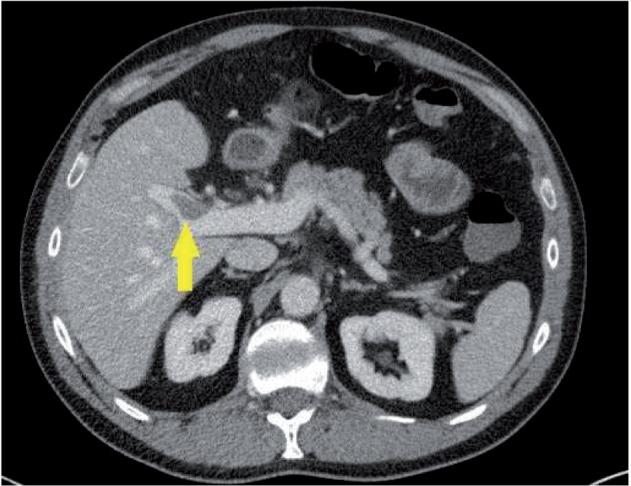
CT of the abdomen, demonstrating presence of thrombus in the portal vasculature.
